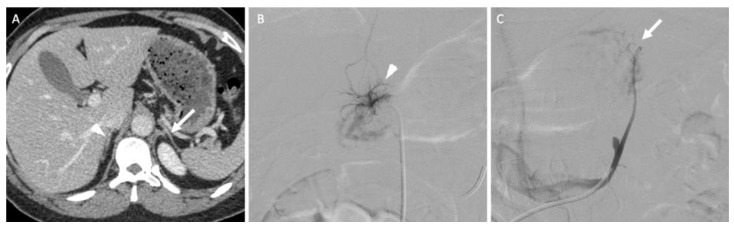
A 45-year-old woman with primary hyperaldosteronism with normal adrenal glands on computed tomography (CT) and MRI who underwent bilateral adrenal vein sampling. (A) The CT image in the axial plane obtained 70 s after intravenous administration of iodinated contrast material shows two thin adrenal glands (the arrow indicates the left adrenal gland; the arrowhead indicates the right adrenal gland) without a nodular portion. (B) The digital subtracted angiographic image shows selective catheterization of the right adrenal vein (arrowhead) using a 5-F Shepherd hook catheter. (C) The digital subtracted angiographic image shows selective catheterization of the left adrenal vein (arrow) using a 5-F left renal catheter. Blood sample analysis confirmed elevated aldosterone secretion from the right adrenal gland. The patient underwent right adrenalectomy, and her aldosterone serum level returned to normal values.
